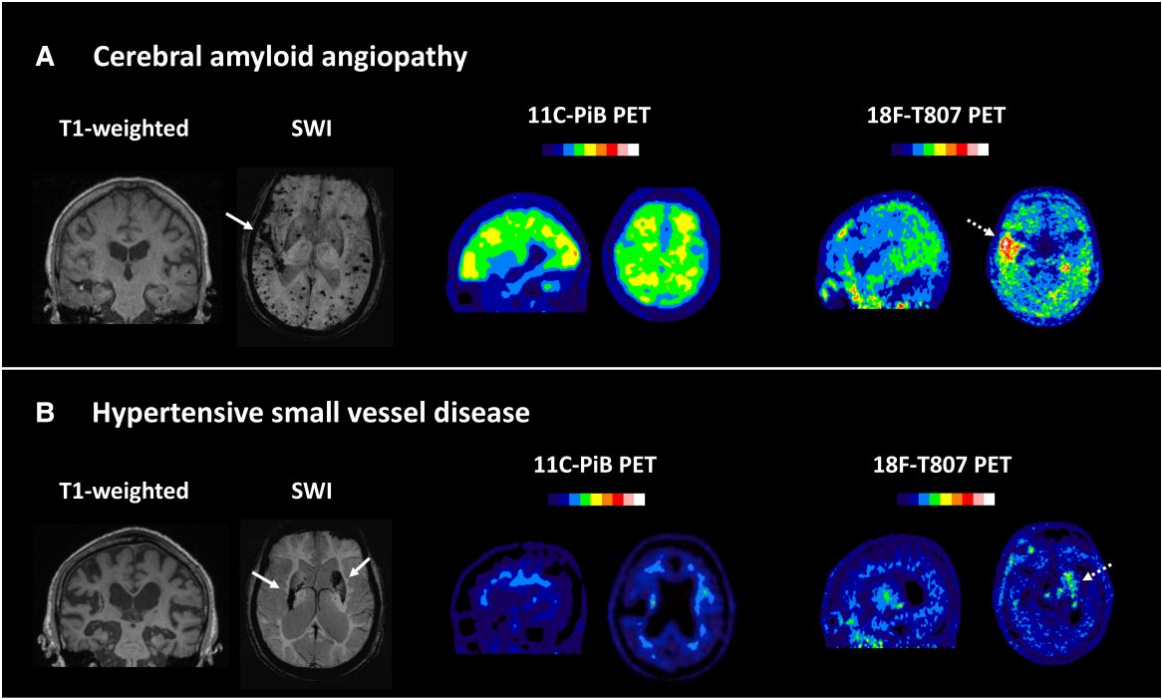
Representative amyloid and tau scans of CAA and HTN-SVD. Representative MRI, PiB PET and tau PET images of (A) CAA and (B) HTN-SVD. Patients with CAA exhibited higher amyloid and tau uptakes compared with patients with HTN-SVD. Off-target binding to haematoma (arrows and dashed arrows) was observed on T807 PET scans.
